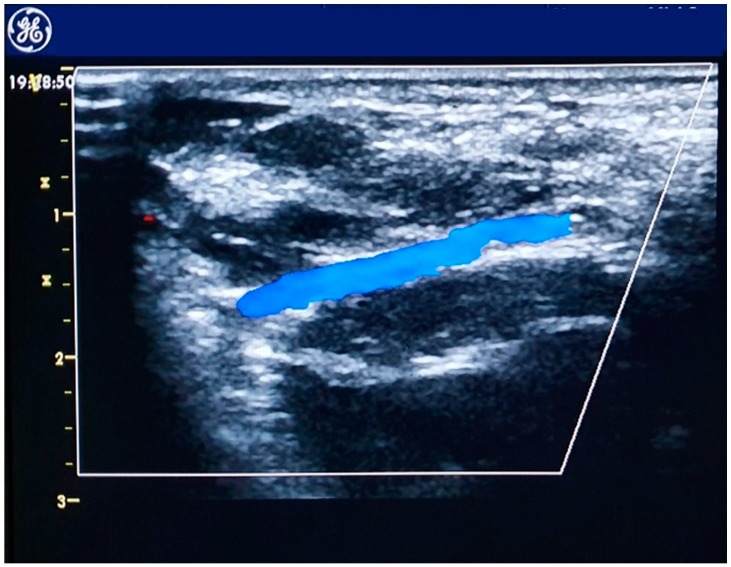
Echocolordoppler image of the distal radial artery.
